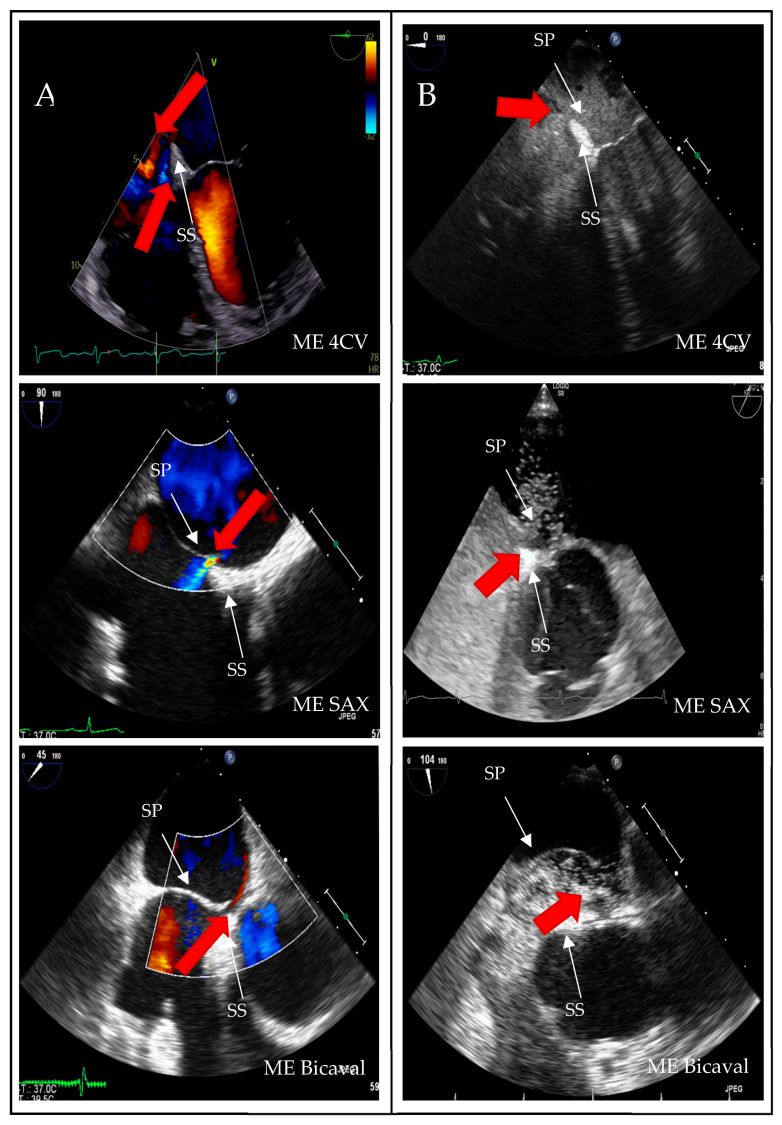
Two-dimensional TOE recordings of an IAS with PFO. Shown is a short circuit between the RA and LA in ME 4CV, ME SAX, ME Bicaval image planes (A) in the color Doppler and (B) in the bubbles test; the red arrow indicates the direction of the short circuit. ME: midesophageal, SAX: short axis, 4CV: four chamber view, SP: septum primum, SS: septum secundum.
